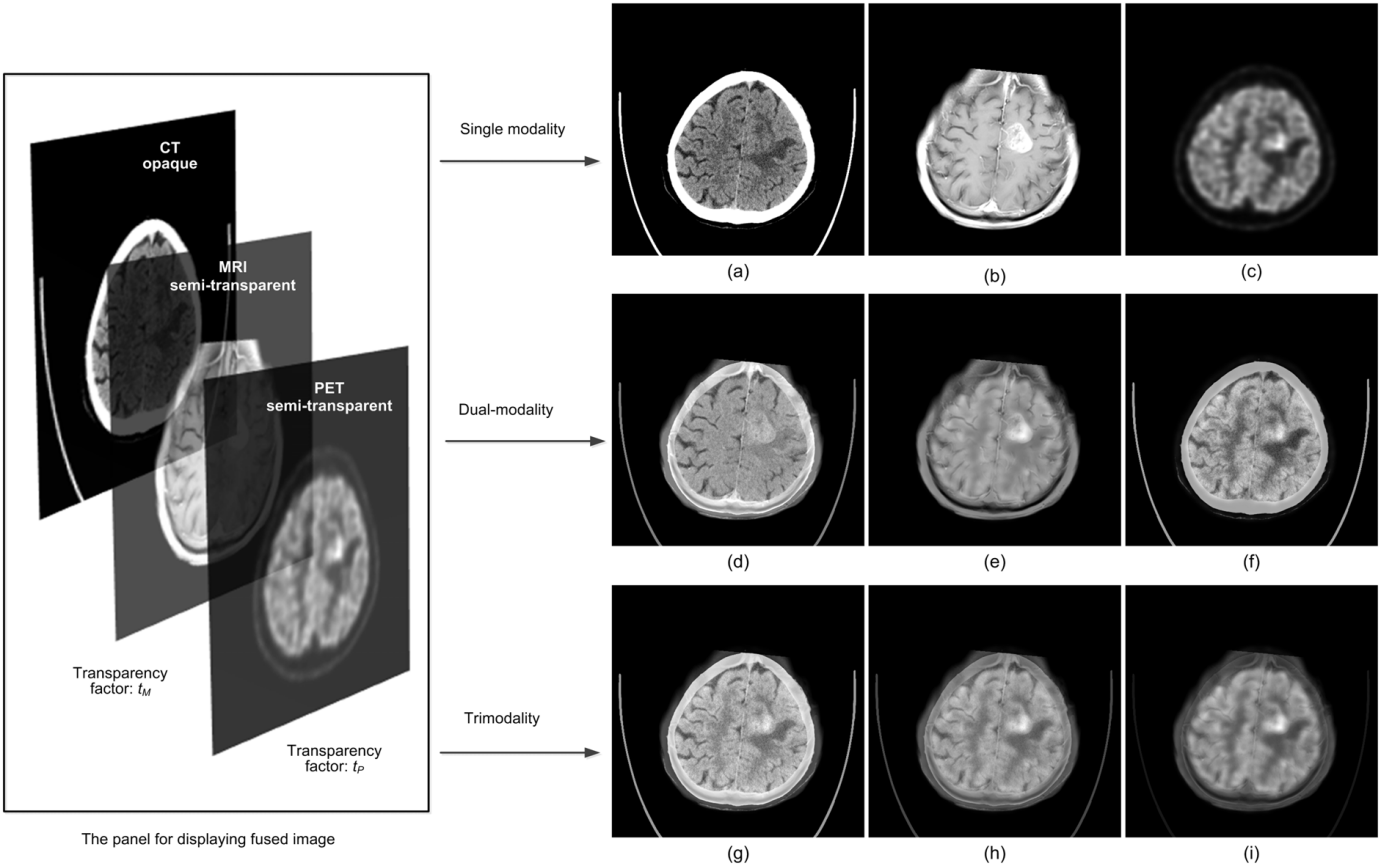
A glioma tumor shown on CT, MRI, and PET images with various *t_P_* and *t_M_* altering in the range of 0 to 1 for patient 1. (a) t_P_ = 0, t_M_ = 0; (b) t_P_ = 0, t_M_ = 1;(c) t_P_ = 1, t_M_ = 0; (d) t_P_ = 0, t_M_ = 0.5; (e) t_P_ = 0.5, t_M_ = 1;(f) t_P_ = 0.5, t_M_ = 0; (g) t_P_ = 0.3, t_M_ = 0.3; (h) t_P_ = 0.5, t_M_ = 0.5; (i) t_P_ = 0.7, t_M_ = 0.7.
